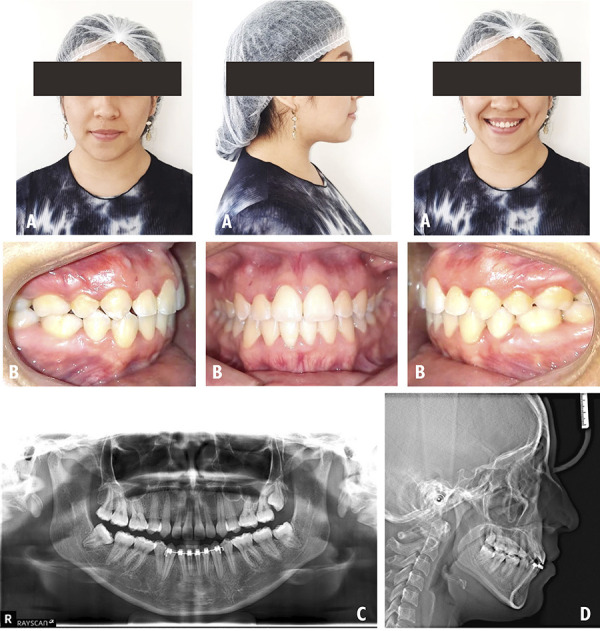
Case 1. Post-treatment records. A: Extraoral photographs. B: Intraoral photographs. C: Panoramic radiograph. D: Cephalometric radiograph.
